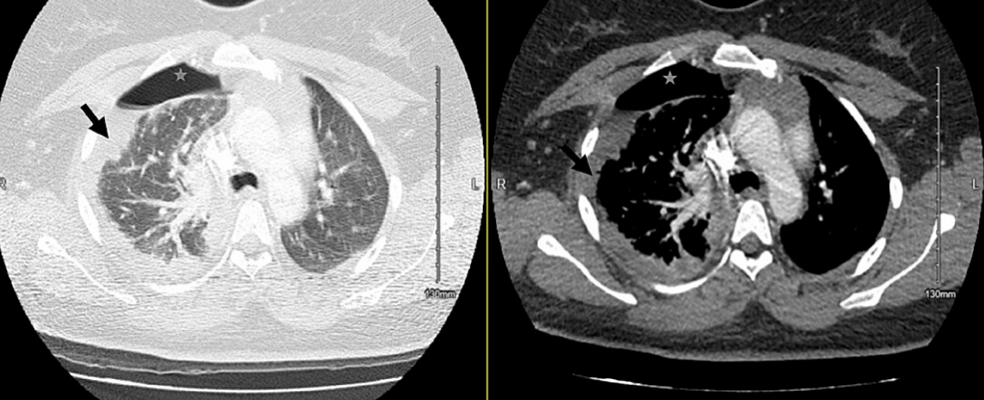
CT scan of chest showing right-sided loculated hydropneumothorax (grey star) and right-sided consolidation (black arrow) CT: computerized tomography
